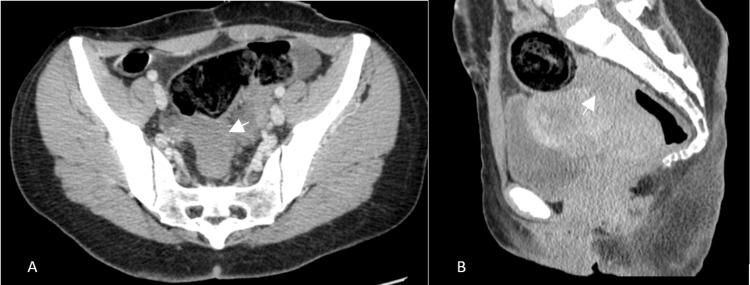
Axial (A) and sagittal (B) pelvic CT images demonstrating a focal area of rectosigmoid wall thickening with transmural contrast enhancement (white arrow), causing luminal stenosis and suggestive of a pseudo-tumoral lesion.
